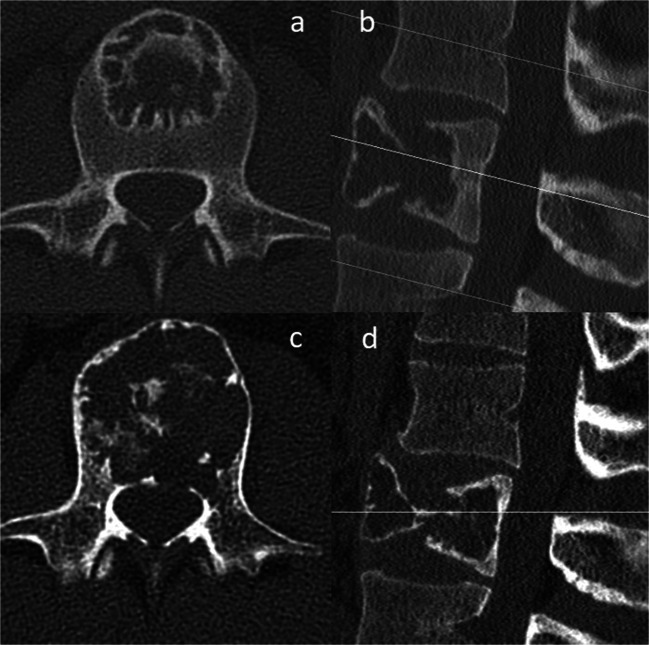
a, b Preoperative sagittal and axial computed tomography (CT) scans (2016) of L2. c, d Progression of the cystic cavitation with involvement of the anterior and posterior edge (2018)
